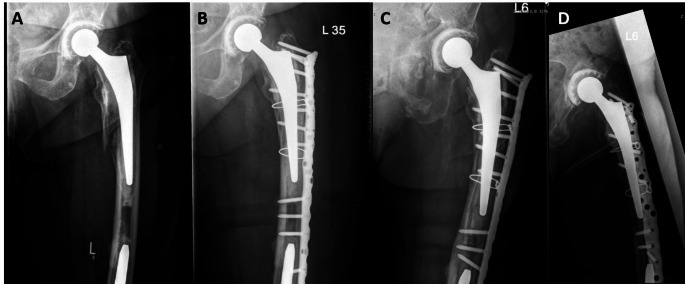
88 years-old female patient with a Vancouver B1 periprosthetic femur fracture who was operated during daytime. A) AP radiograph of the left hip after fall from standing height. B) AP radiograph at postoperative day 2 after locking plate fixation. C) + D) AP and axial radiograph 2 years postoperatively showing a healed fracture
